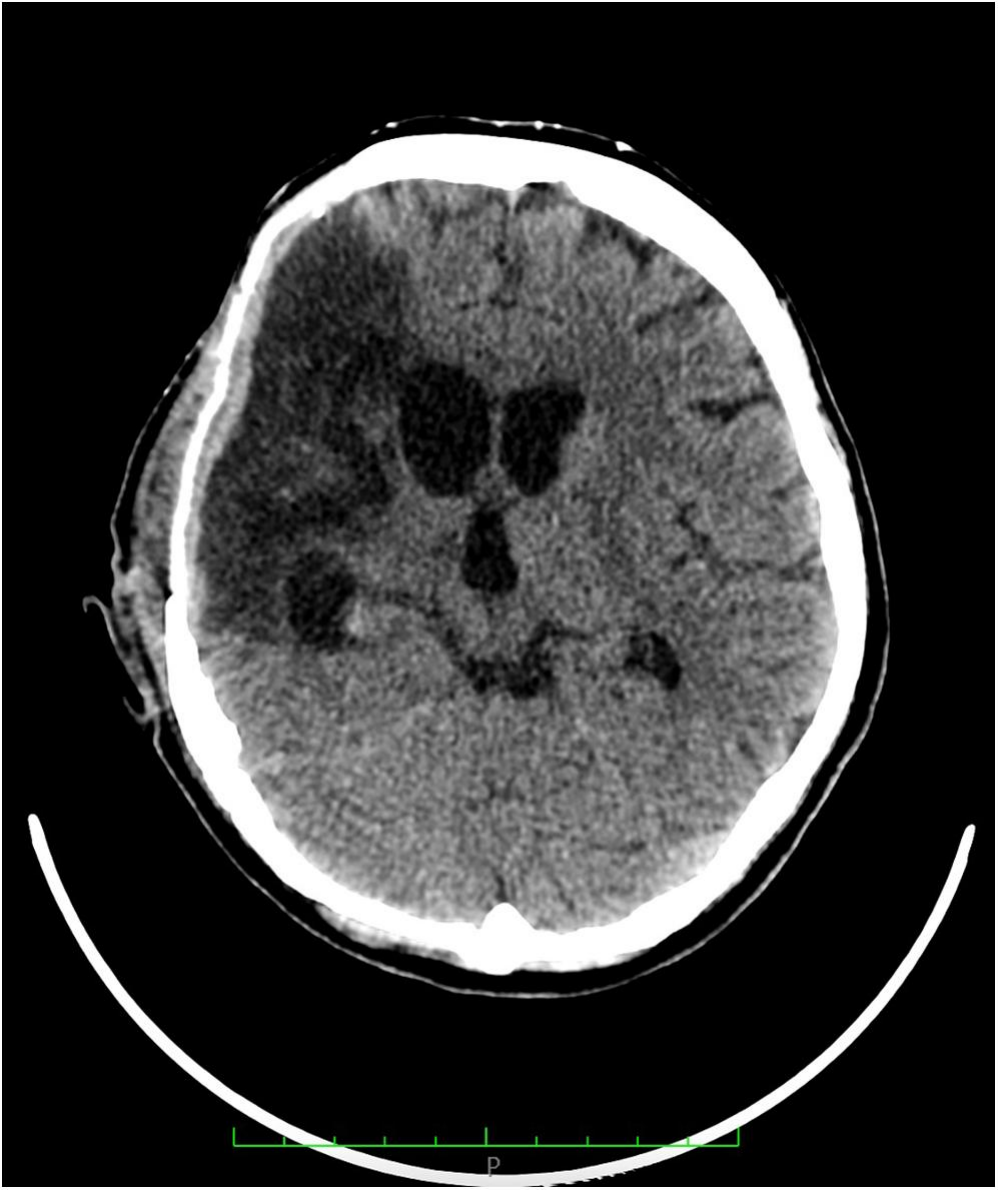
Post‐operative head CT scan showing the correct placement of the cranioplasty
